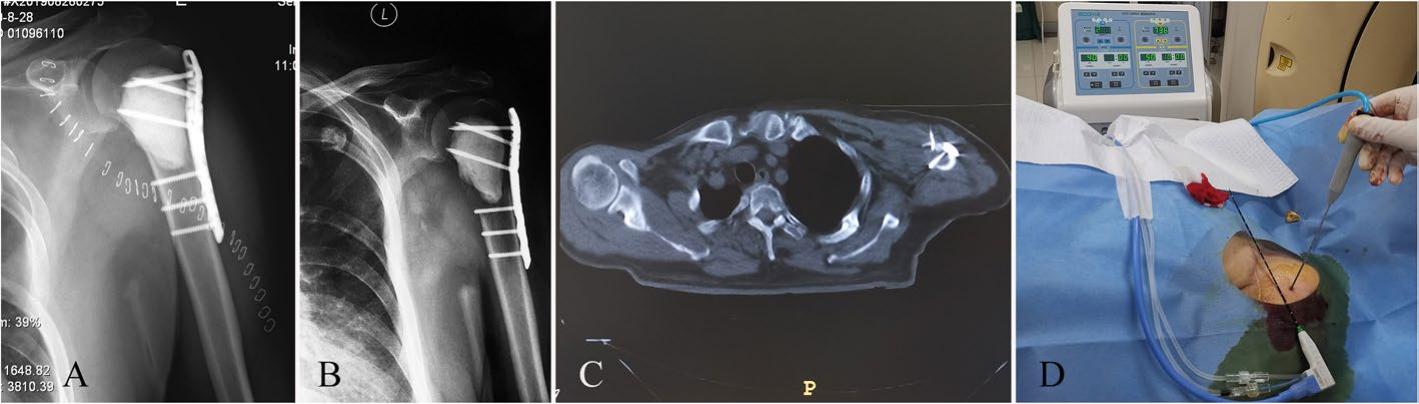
A 63-year-old male patient with ﻿with recurrent bone metastasis in the proximal humerus treated by percutaneous microwave ablation. A X-ray after the first surgery shows bone metastasis curettage, cementation and internal fixation. B X-ray at postoperative 8 months shows recurrent bone metastasis under the cement. C Percutaneous microwave ablation has been used to treat the recurrent bone metastasis. D After microwave ablation of the tumor, the temperature of the ablation core area can be measured to 73 ℃
